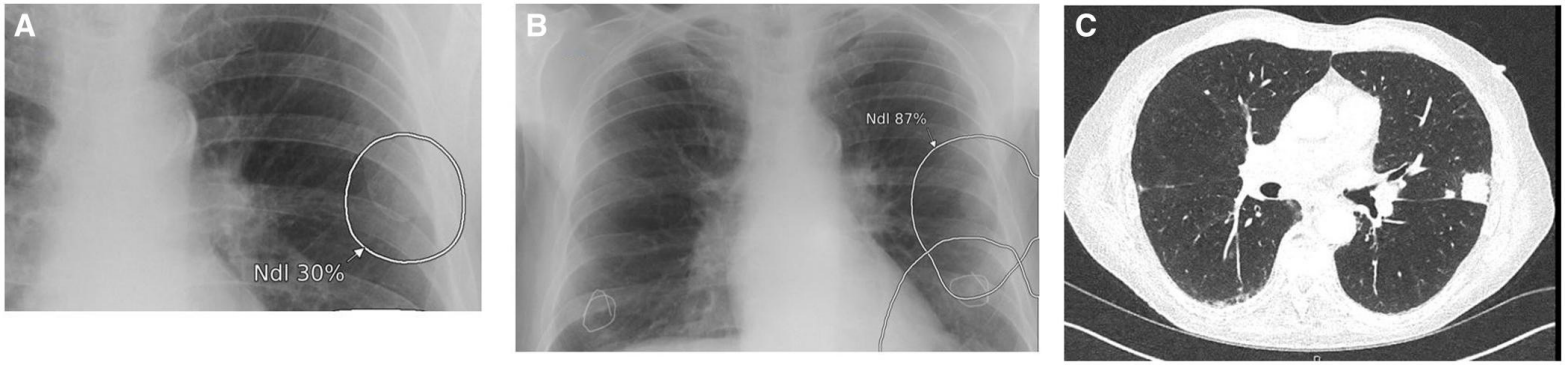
An 85-year-old male with a history of productive cough and rigors underwent a CXR (A), reported as NAD. The AI software demonstrates suspicion of a nodule on the left side of the chest periphery, behind the scapula, and ribs. Six months later, the patient returns for another CXR (B) due to being unwell, whereby the report and AI software are suspicious of a nodule on the left side. Note that the scapula is removed from the lung field, making it easier to visualize the nodule, also note nipple markers on the image (B). A CT scan is subsequently performed (C) demonstrating a left-sided mass confirmed as stage 3b LC. AI = artificial intelligence; CXR = chest radiograph; LC = lung cancer; NAD = no abnormility detected.
